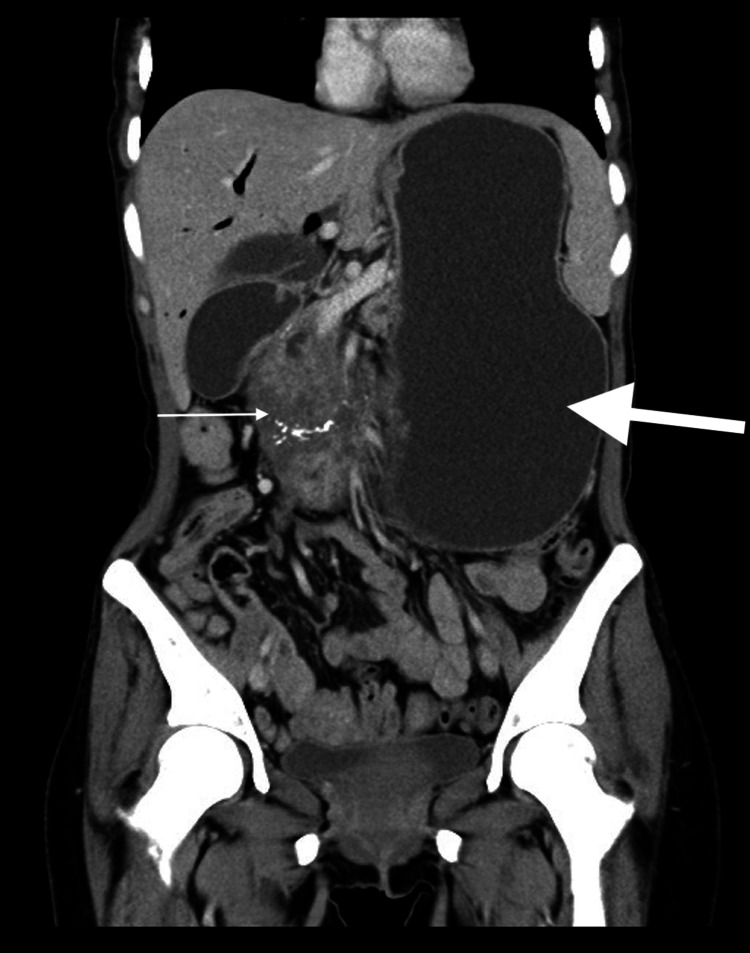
Coronal reformat image of the abdomen CT scan showing a mass in the head of the pancreas (thin arrow) and massive gastric dilatation (thick arrow)
